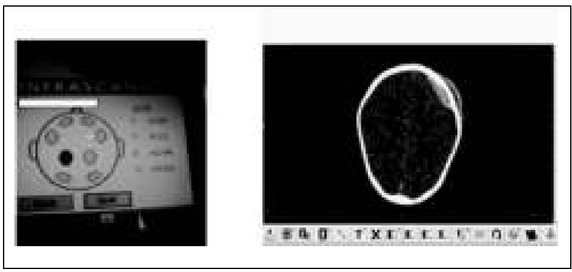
Pozytywny wynik Infraskannera. W TK głowy w okolicy ciemieniowo-czołowej lewej krwiak nadtwardówkowy podostry z cechami niewielkiej progresji (materiał własny). Fig. 3. ositive Infrascanner screening result. Head CT revealed subacute epidural haematoma with slight progression evidence in the left parietofrontal region (own material).
